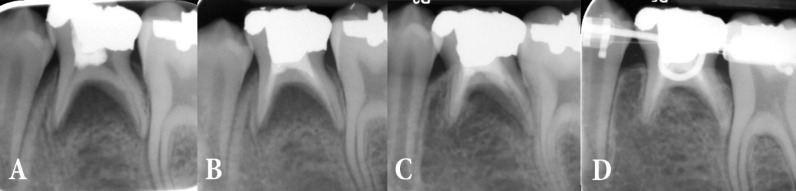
A)* Pre-operative radiograph of the mandibular second primary molar with previous pulpotomy; note the missing of permanent second premolar; *B)* Immediate post-endodontic treatment radiograph of the tooth and root filling with CEM cement; *C)* Six-month follow-up radiography; *D)* After 12 months the tooth is asymptomatic and fully functional; note the complete inter-radicular bone healing
